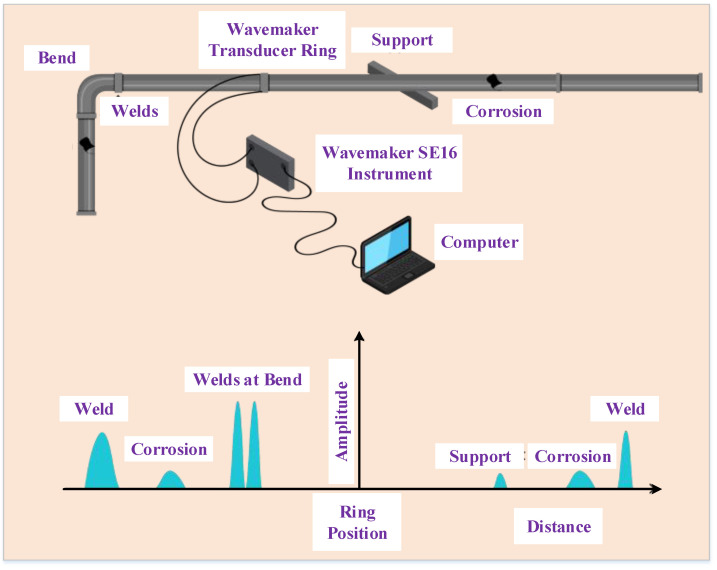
Ultrasonic monitoring for corrosion assessments adopted from [55].
